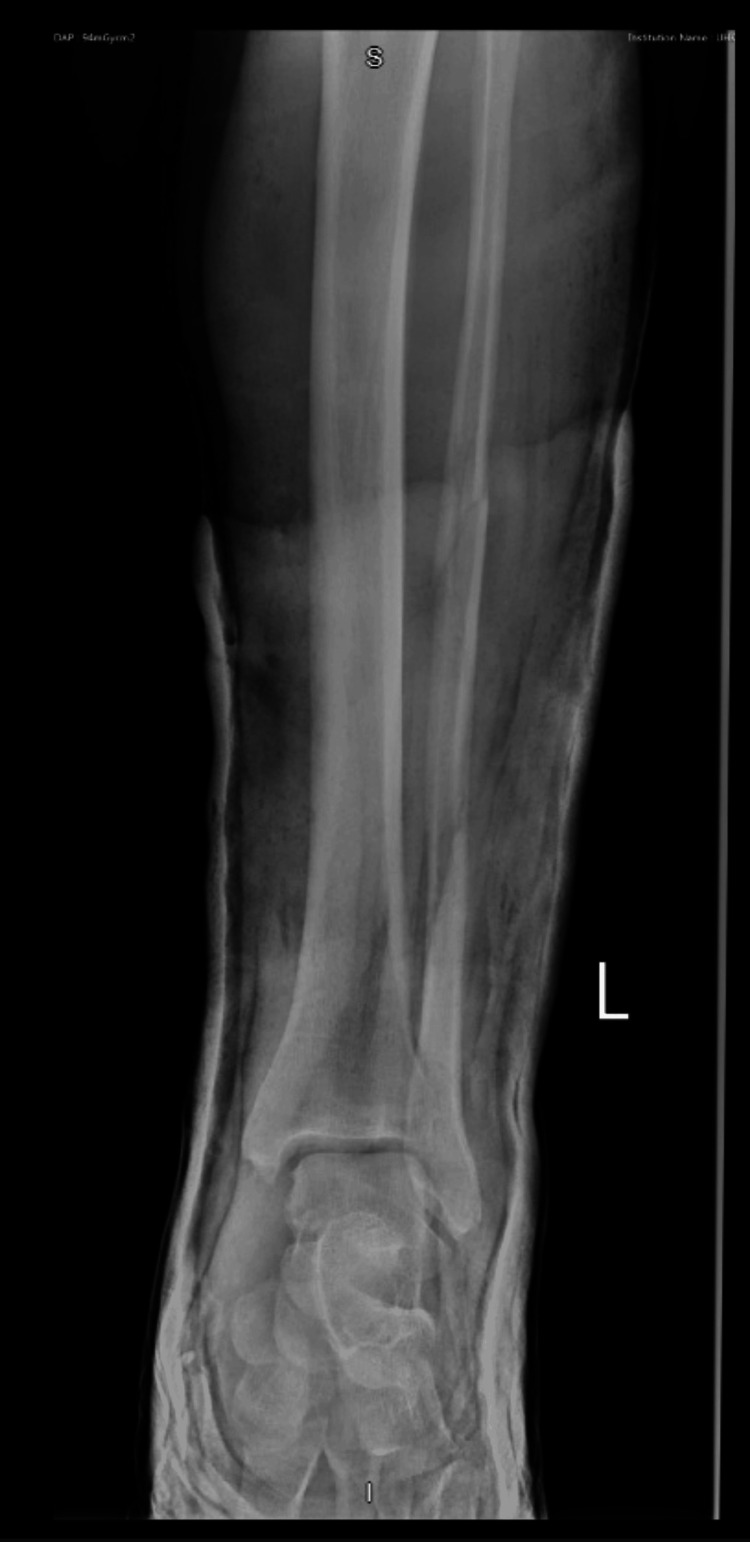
Anteroposterior left ankle radiograph post-reduction under conscious sedation and application of a backslab
